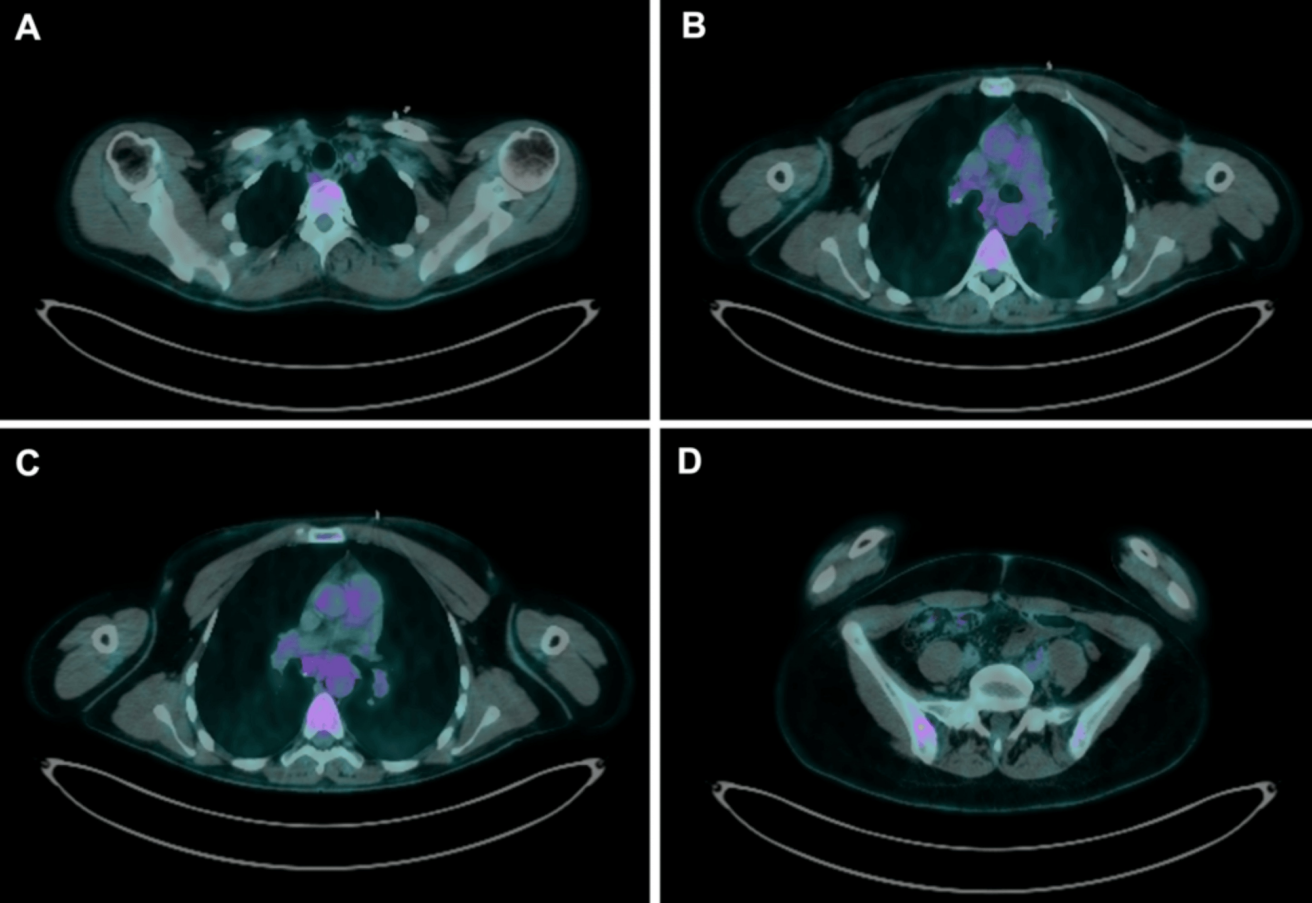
Follow-up ¹⁸F-FDG PET/CT images showing (A) resolution of pulmonary uptake, (B, C) mild residual metabolic activity in mediastinal and hilar lymph nodes (SUVmax = 3.1), and (D) moderate uptake in the right iliac bone (SUVmax = 4.3). ¹⁸F-FDG PET/CT: fluorine-18 fluorodeoxyglucose positron emission tomography/computed tomography
